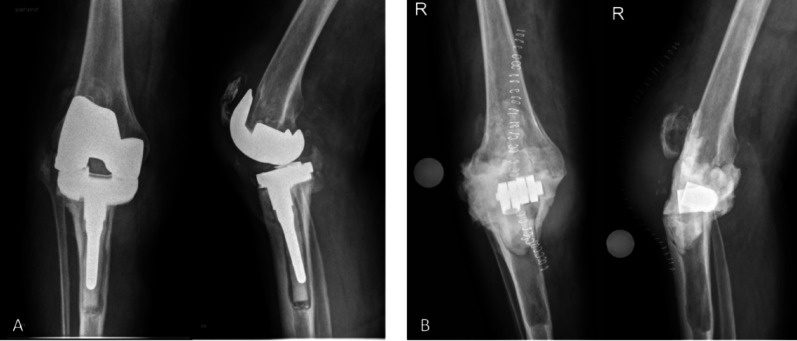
A Preoperative radiographs demonstrating septic loosening of a total knee arthroplasty. B Postoperative radiograph after prosthesis removal and implantation of an arthrodesis bone cement spacer
